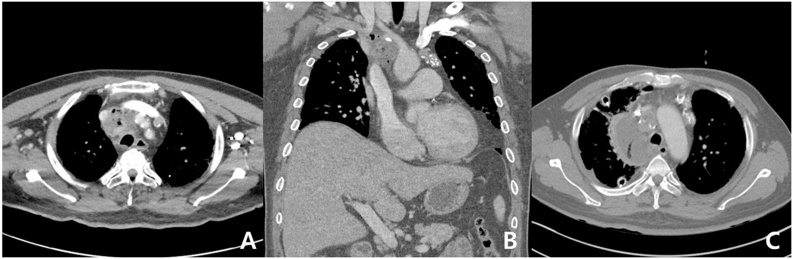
(A, B) Chest CT, performed 21 days after EBUS-TBNA, shows enlargement of the lymph node accompanied by the intranodal necrotic portion; (C) chest CT, performed 5 days after operation, shows loculated effusion with newly generated gas formation.
